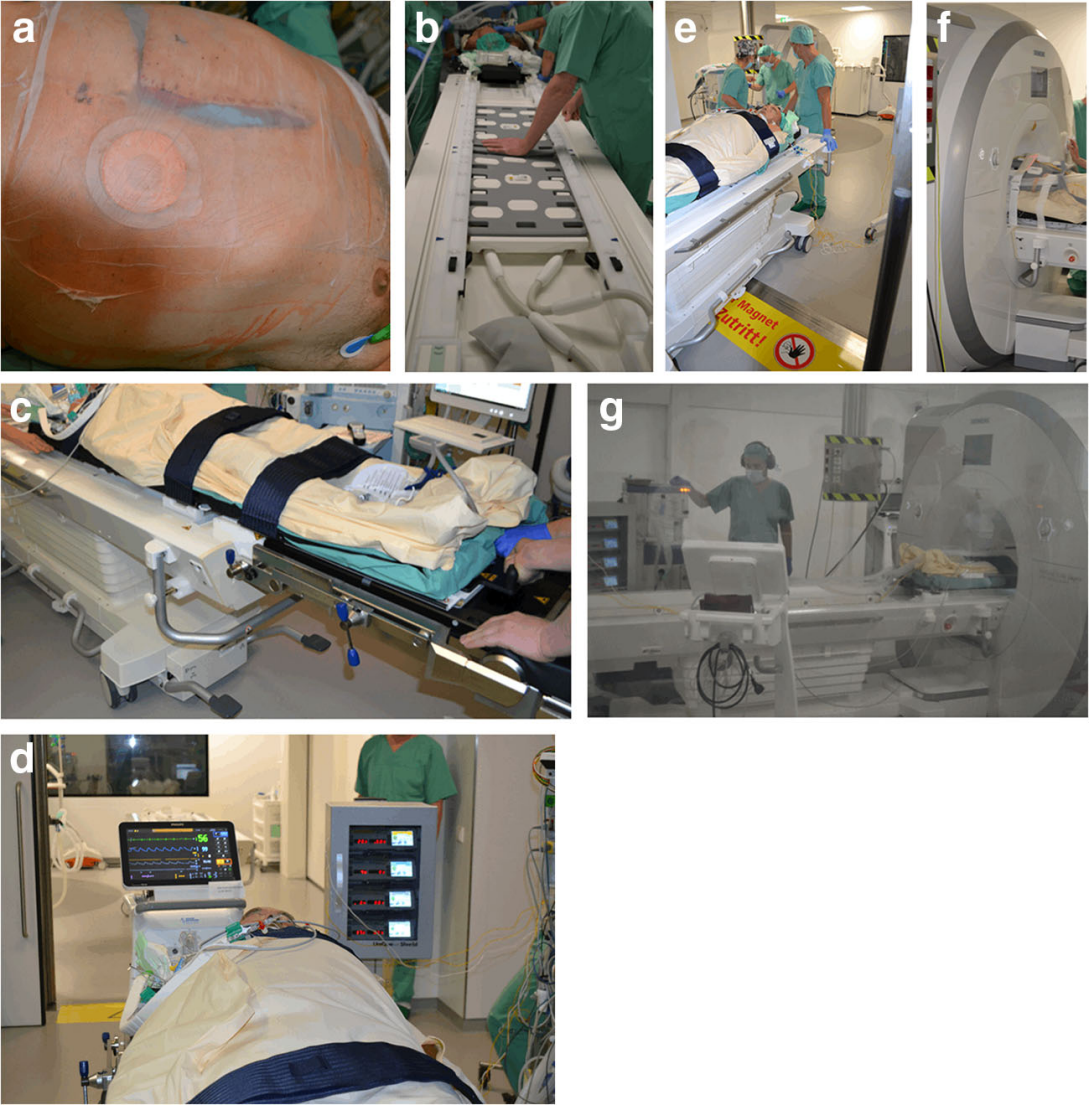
a Temporary closure of the abdominal wall by abdominal linen and self-adhesive drape. b and c Patients transfer from OR table to MRI table by using a special mobile transfer board. d View of the OR towards the connected MRI suite showing special MRI-compatibel anesthesiological equipment. e and f Patients’ transfer into the MRI suite (e) and positioning in the MRI with phased array body coil (f). g View from the MRI control room: anesthesiologist performing apnea episodes during ioMRI
